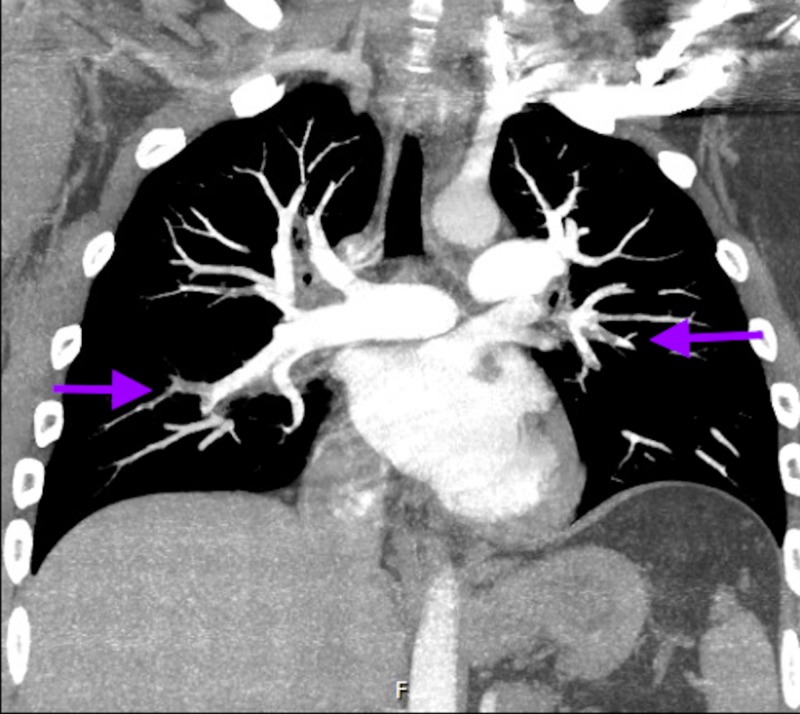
Computed tomography angiography (CTA) of the chest showing subsegmental embolism
